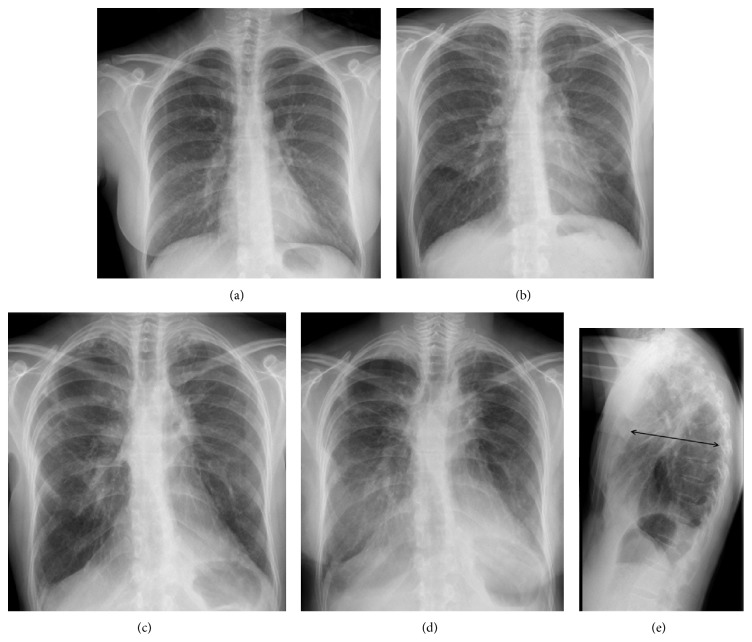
Case  2. (a) Initial chest radiograph after the diagnosis of breast cancer and before chemotherapy and radiotherapy treatment was normal. (b), (c), and (d) Chest radiographs evolution along two-year and two-month interval reveal progressive worsening. Notice progressive gradual loss of lung volume at the expense of the upper lobes, which is manifested by superior shrinkage and distortion of the pulmonary hila, as well as biapical pleural thickening. (e) Lateral chest radiograph shows flattening of the chest, observed by the decreased anteroposterior diameter of the rib cage (arrow).
